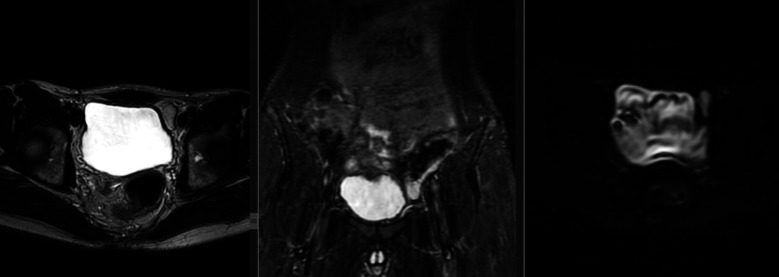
MRI localized testis in left low intra - abdominal location, as shown on (a) axial T2W, (b) coronal T2W fat - sat, and (c) axial DWI.
